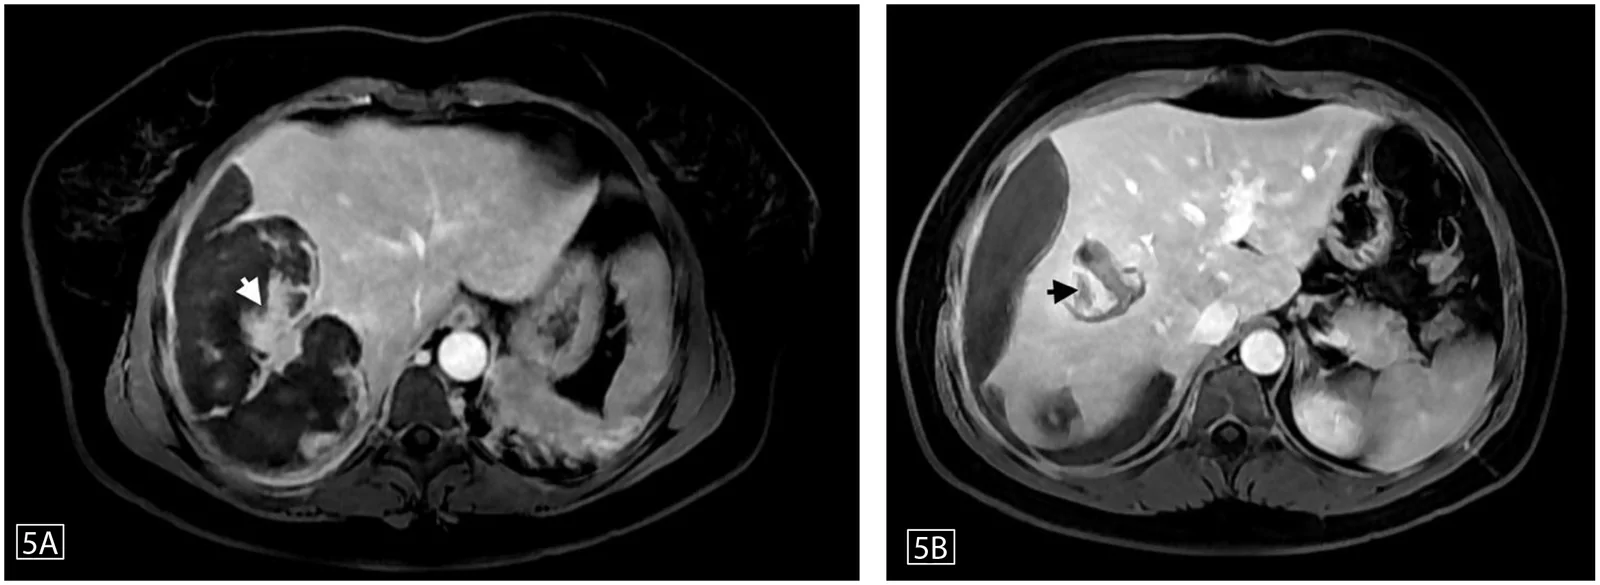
Dynamic MRI demonstrating arterial enhancement in 2 distinct hepatocellular adenomas. (A) Arterial-phase MRI showing heterogeneous enhancement within the viable components of the large hemorrhagic adenoma involving segments VII and VIII (white arrowhead). (B) Arterial-phase MRI demonstrating similar enhancement within the separate 3-cm adenoma located in segment V (black arrow).
